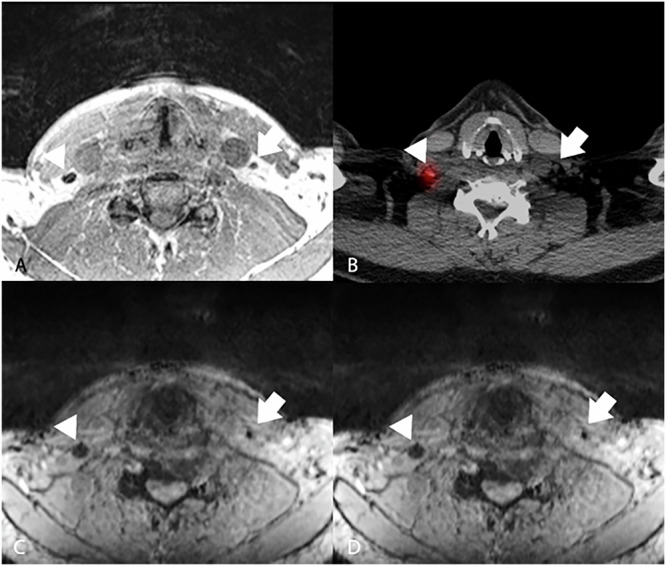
Transversal images of patient #6 with a right-sided lateralized tongue tumor after injection of 0.036 mL of SPIO. A T1-weighted VIBE Dixon, in-phase image; B fused SPECT/CT; T2*-weighted images computed TE of 5 ms (C) and 10 ms (D). Arrowheads indicate ipsilateral drainage of both ICG-[^99m^Tc]Tc-nanocolloid and SPIO on SPECT/CT and MRI. Arrows indicate contralateral drainage of SPIO, whereas no drainage of ICG-[^99m^Tc]Tc-nanocolloid is seen on the SPECT/CT. ICG, Indocyanine green; MRI, Magnetic resonance imaging; SPECT/CT, Single-photon emission computed tomography/computed tomography; SPIO, Small particle iron oxide; TE, Echo time; VIBE, Volume interpolated gradient-echo
